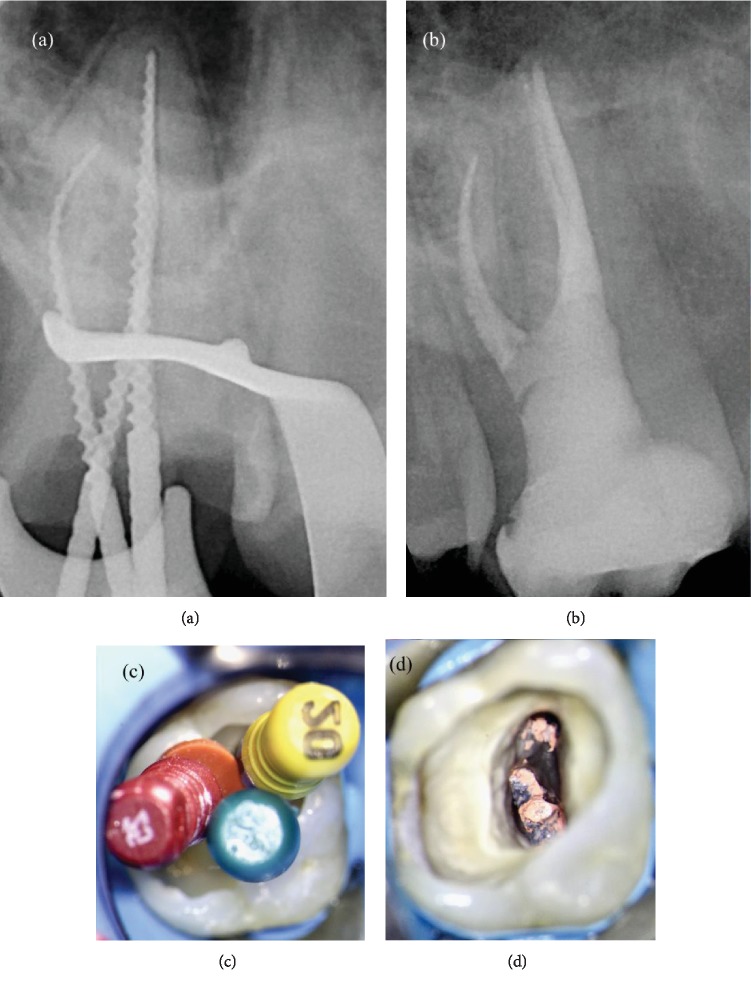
(a) Intraoperative orthoradial X-ray using verifiers. (b) Postoperative angulated X-ray. (c) Intraoperative photo with verifier. (d) Intraoperative photos after Thermafil filling.
